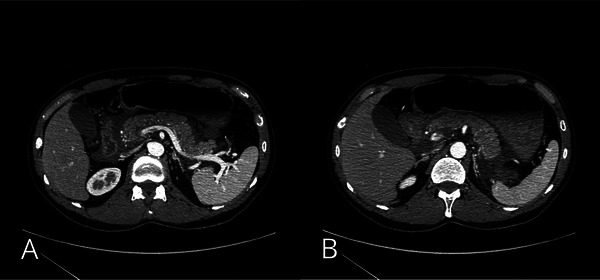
(A) Contrast‐enhanced computed tomography showing increased fat stranding around the pancreas. (B) The duodenum appears edematous, and gastric distension is observed.
